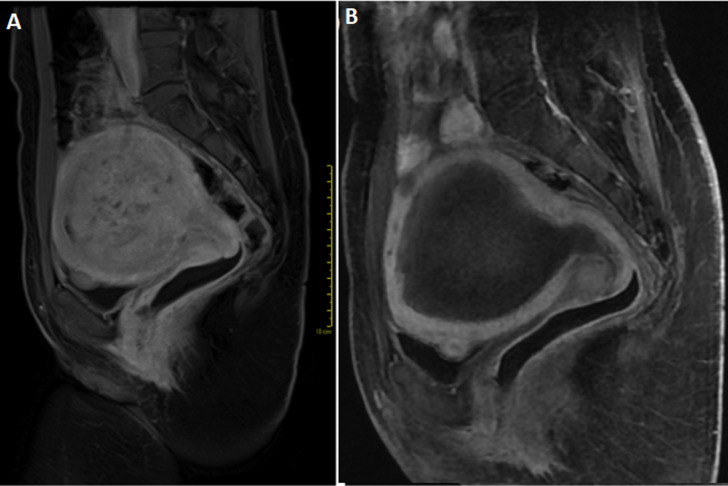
A) MRI before embolization (sagittal post contrast T1 fat saturation image demonstrated intense contrast enhancement of the myoma); B) MRI after embolization (sagittal post contrast T1 fat saturation image); it was observed that the myoma was no longer contrast-enhanced after embolization and its dimensions decreased
